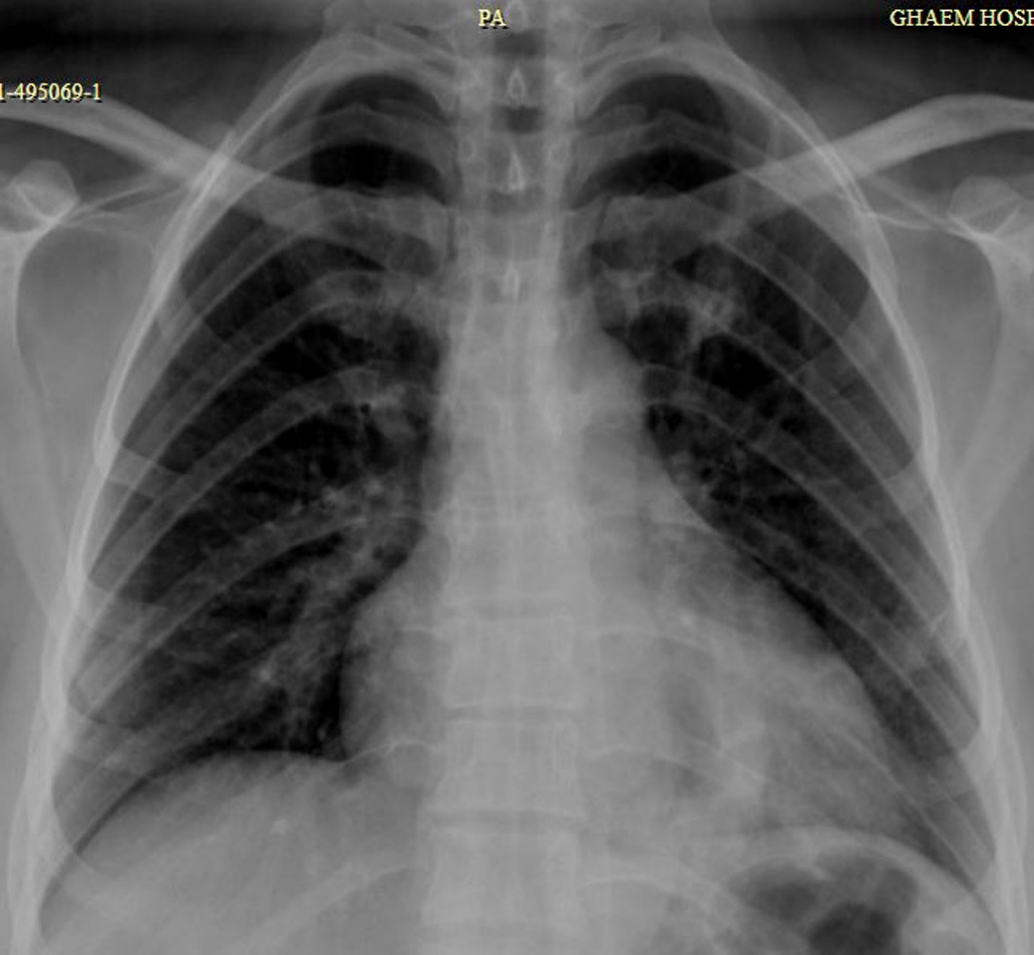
PA chest radiograph shows which does not reveal any mediastinal widening or other pathological findings.
